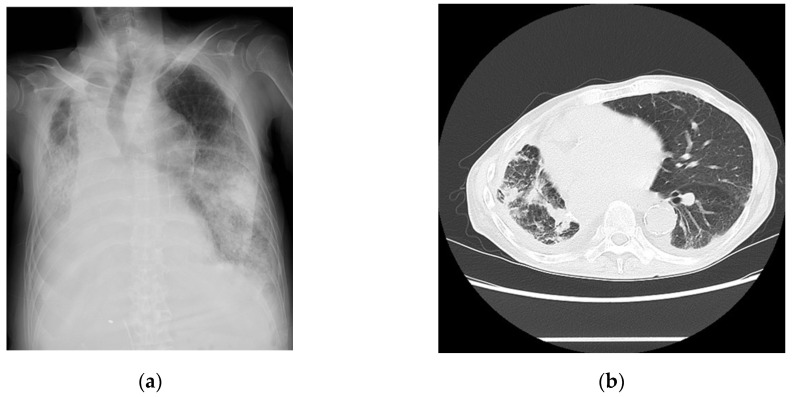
Imaging findings for Case 3 captured on day of admission. (a) Chest radiograph and (b) chest computed tomography image showing bilateral extensive findings of pneumonia with air bronchogram.
